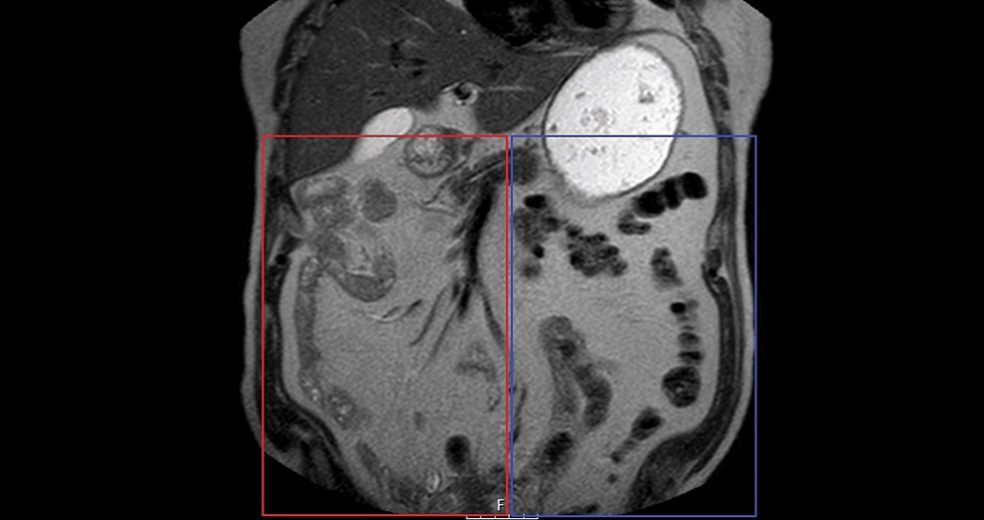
Coronal MRI showing the small intestine positioned on the right side of the abdominal compartment (red square), in contrast to the left-sided position of the colon (blue square).
